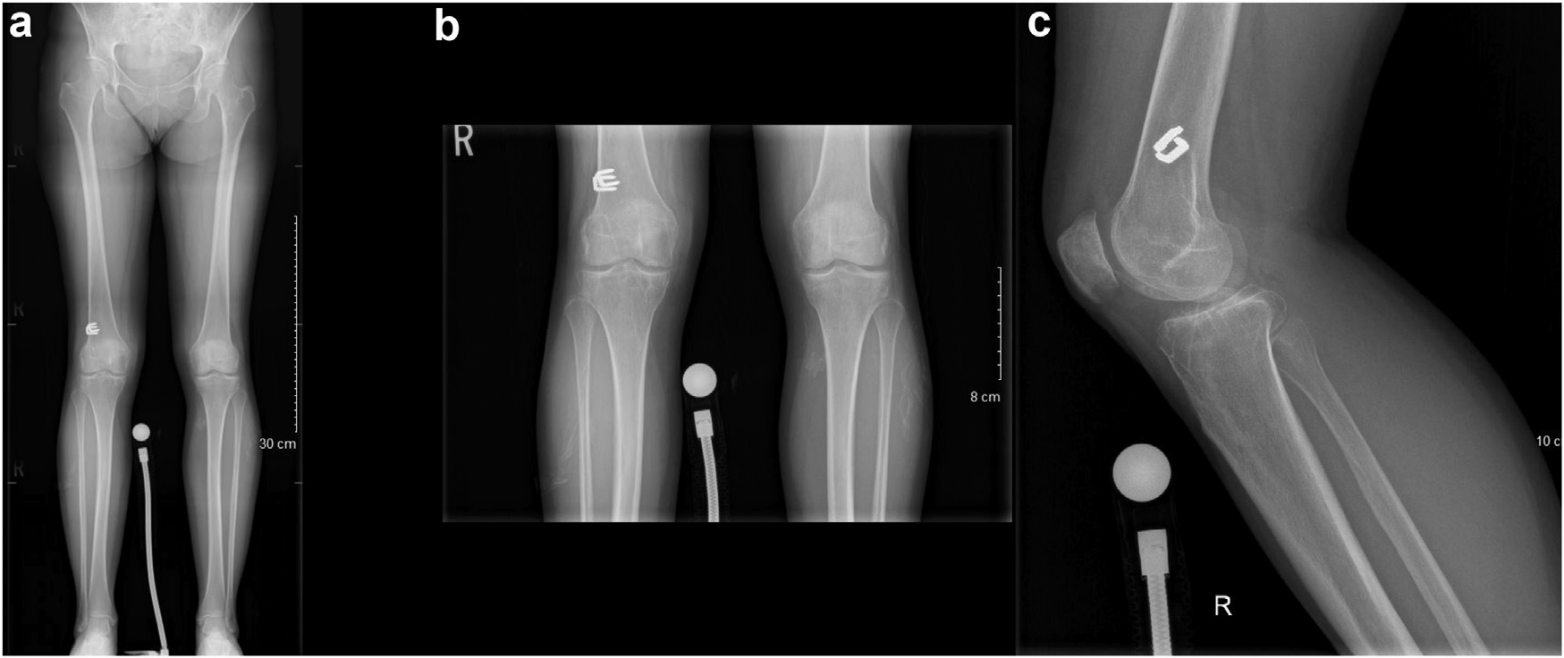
Preoperative images: (a) full-length standing antero-posterior X-rays of both lower extremities; (b) antero-posterior x-rays of both knees; and (c) lateral of the right knee. The anterior cruciate ligament (ACL) staples of the previous ACL reconstruction are visible on all 3 views.
